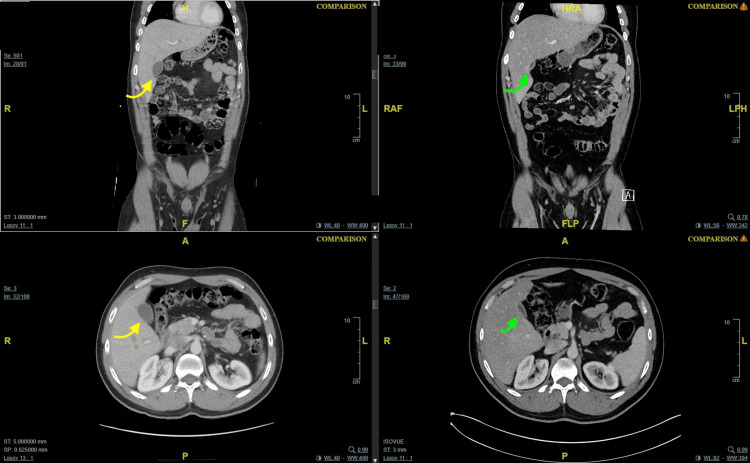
CT scan of abdomen and pelvis with a repeat in three years. The left upper and bottom panels represent the prior CT scans showing a distended non-inflamed gallbladder (yellow arrows). The right upper and bottom panels represent the latter CT scan showing a constricted non-inflammed gallbladder (green arrows). Both show no gallstones, wall thickening, or pericholecystic fluid suggesting acute cholecystitis.
